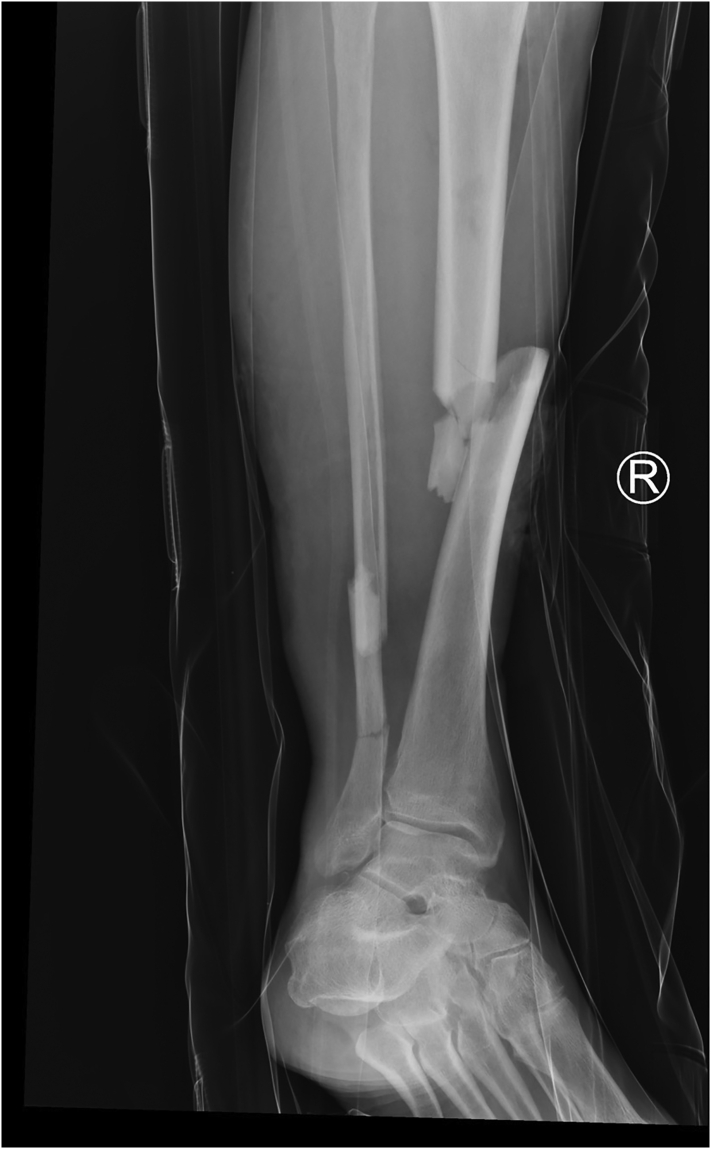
Anterior posterior radiograph of the right leg showing a comminuted midshaft fracture of the tibia with a minimally displaced comminuted fracture of the fibula.
